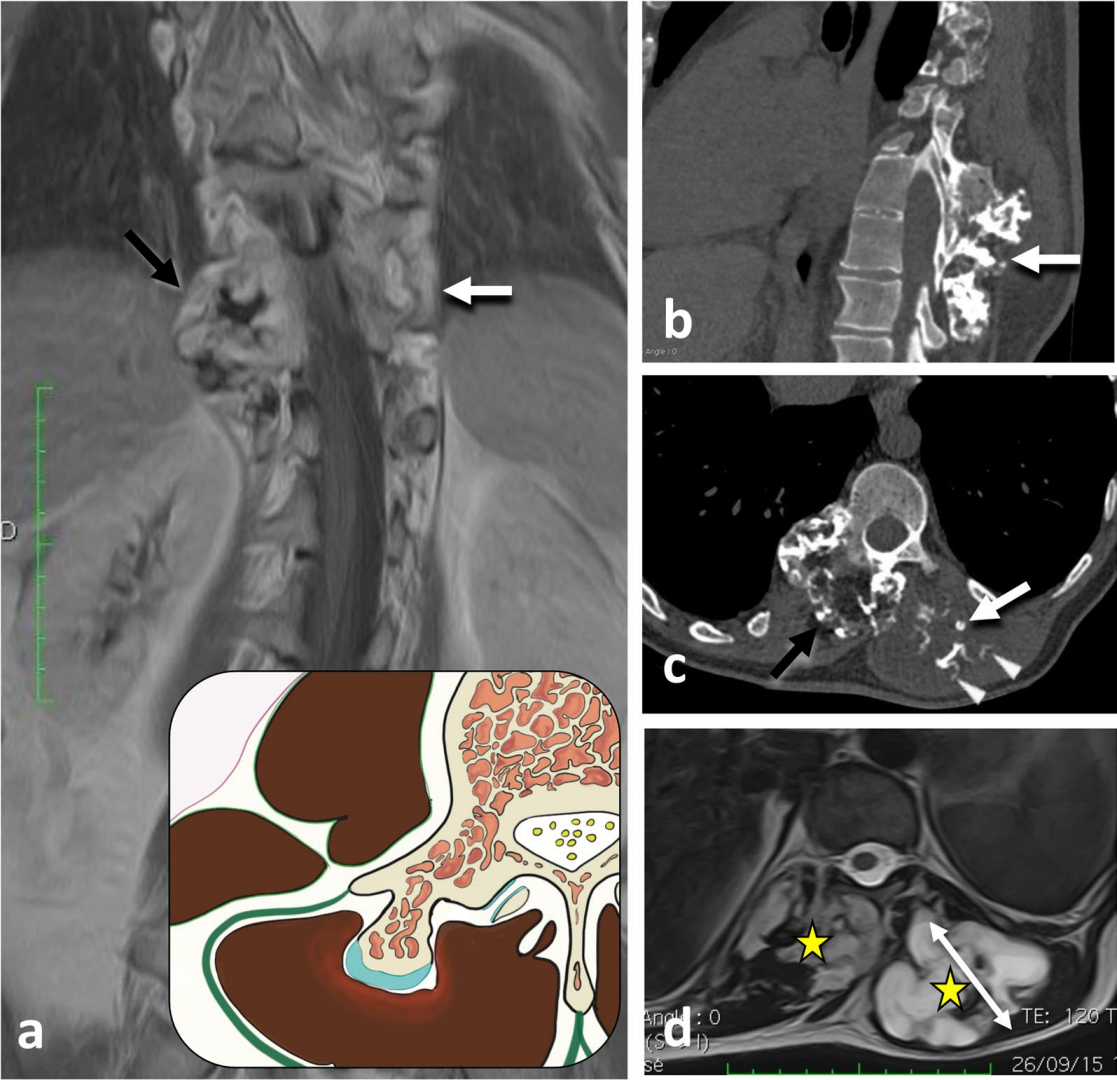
Exostosis and chondrosarcoma*, respectively, on the right and on the left transverse processes of T10 in a 57-year-old male with hereditary multiple exostosis. The patient had been experiencing back pain for 2 months, a possible indicator of malignant degeneration of a chondroma into a chondrosarcoma. The patient presents with a right osseous outgrowth (black arrows), which has cortical and medullary continuity with the underlying bone as shown in coronal T1WI (a) and axial CT scan reconstruction (c). The cartilage cap of both exostosis and chondrosarcoma appears with intermediate signal on T1WI (a) and high signal on T2WI (d) and has ring and arc calcifications (arrowheads). The patient presents a left osseous outgrowth (white arrows), with rough cortex and cortical disruption (b, c) and with thick cartilage cap (3 cm, double arrow) on axial T2WI (d). Yellow stars show the epicentre of masses
